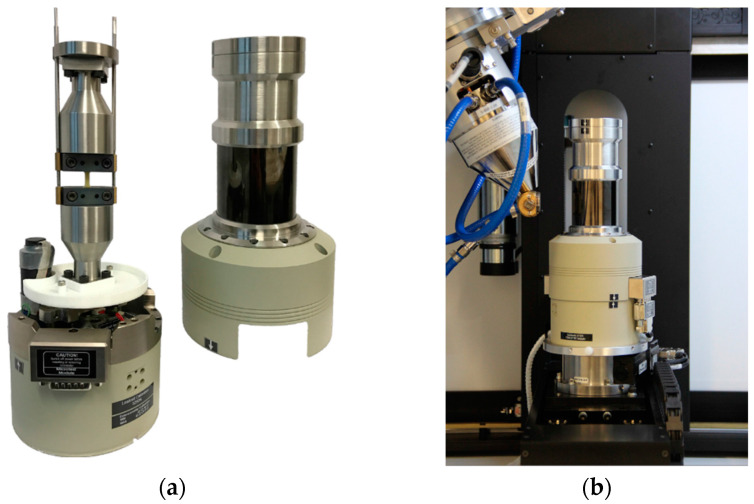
(a) In situ CT testing stage (b) implemented in CT chamber.
